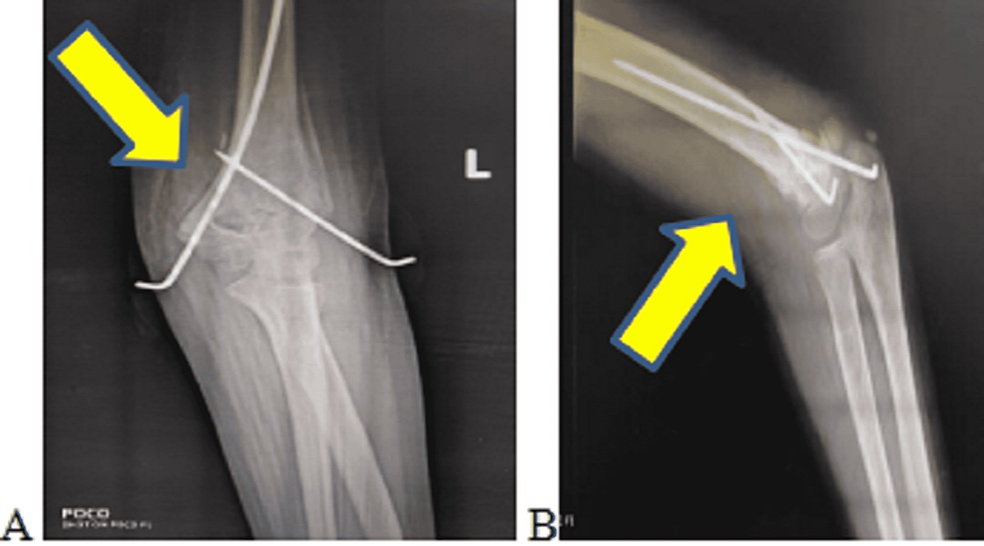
Plain radiographs of the left elbow (A) antero-posterior view and (B) lateral view showing Kirschner wire fixation for left supracondylar fracture with gross rotational anterolateral displacement
